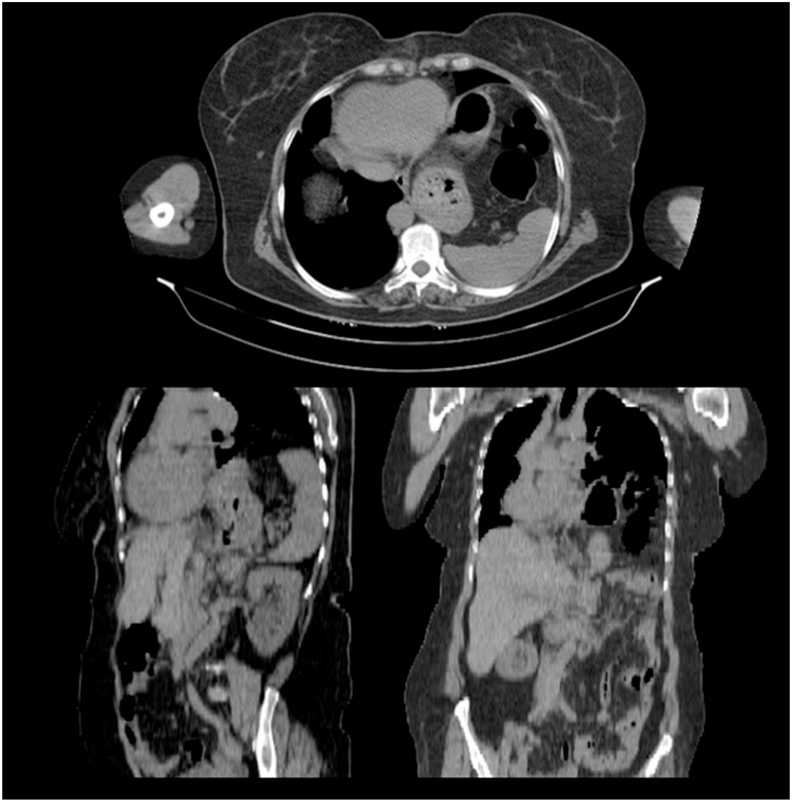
Axial CT scan at the above corroborating the x-ray founds. Accordingly, there are sagittal and coronal reconstructions at the lower left and the lower right of the picture.
